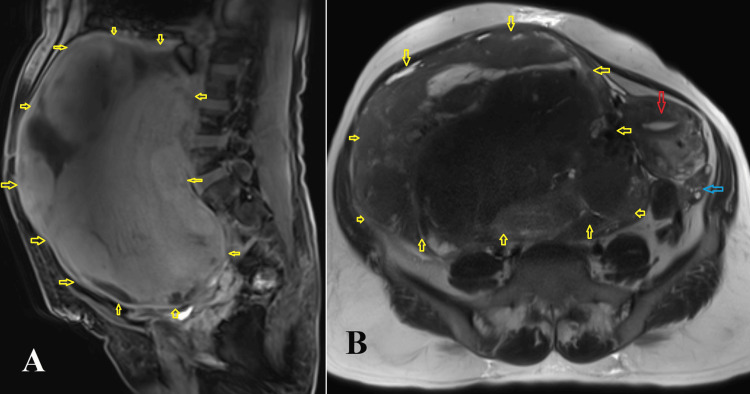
MRI of the abdomen. (A) The sagittal T1 post-contrast scan shows a well-defined mass lesion involving the entire peritoneal cavity extending from the dome of the diaphragm above to the pelvis below. It shows heterogenous enhancement on post-contrast scan. (B) Axial T2-weighted scan shows a large T2 hetero-intense well-defined mass lesion in the right adnexa which is displacing the uterus towards the left side with a well-defined fat plane. Anteriorly, it is abutting the anterior abdominal wall. Posteriorly, it is abutting the lumbosacral spine The yellow arrow marks the tumor, the red arrow marks the uterus, and the blue arrow marks the left ovary. MRI: magnetic resonance imaging
